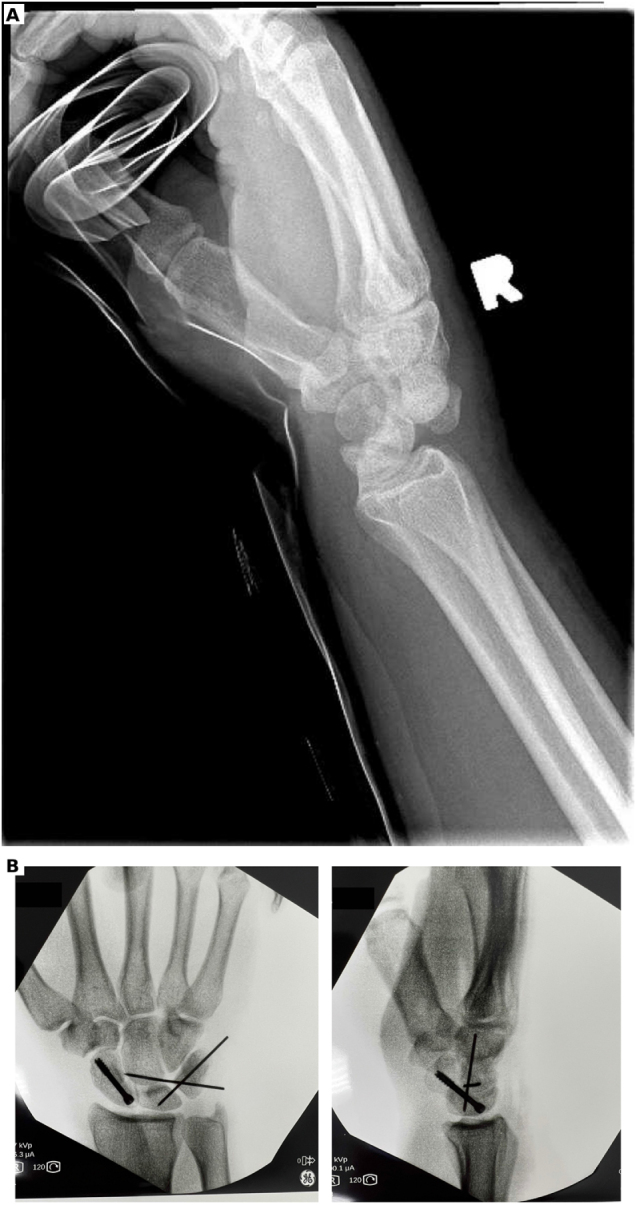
(A) (Case 2, initial injury – pre-reduction): Lateral radiograph of the right wrist demonstrating a perilunate fracture-dislocation with dorsal displacement of the capitate/carpus relative to the lunate and an associated scaphoid fracture at the junction of the proximal and middle thirds. (B) (Case 2, immediate postoperative/intraoperative fluoroscopy): Anterior-posterior (AP) and lateral fluoroscopic images of the right wrist demonstrating anatomic reduction of the scaphoid fracture with headless compression screw fixation, restored carpal alignment, and temporary K-wire stabilization across the lunotriquetral and midcarpal joints.
